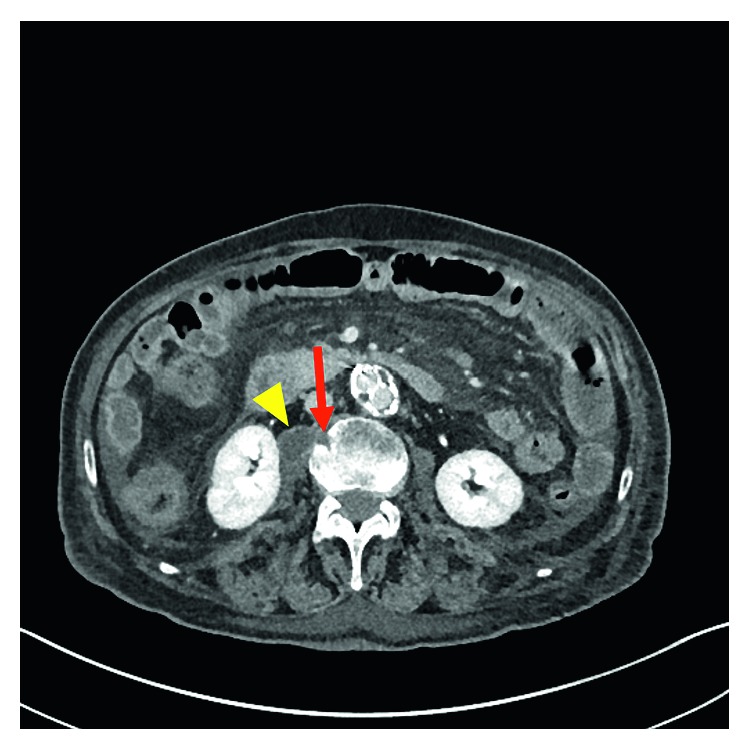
Axial IV contrast-enhanced CT with a small erosion of L2 (red arrow) consistent with osteomyelitis, adjacent to the right psoas abscess (yellow arrowhead).
